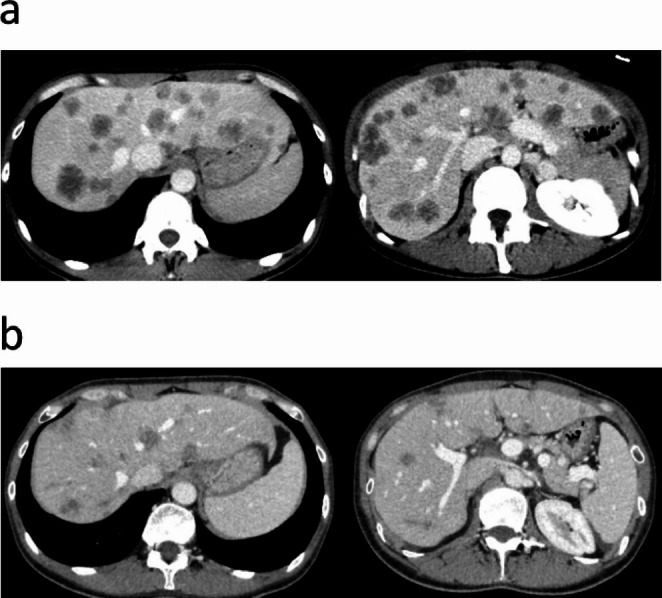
Computed tomography (CT) images before (a) and after (b) systemic chemotherapy of a patient who underwent liver transplantation for unresectable colorectal liver metastases. The size of the tumors decreased remarkably after chemotherapy, but remained unresectable
